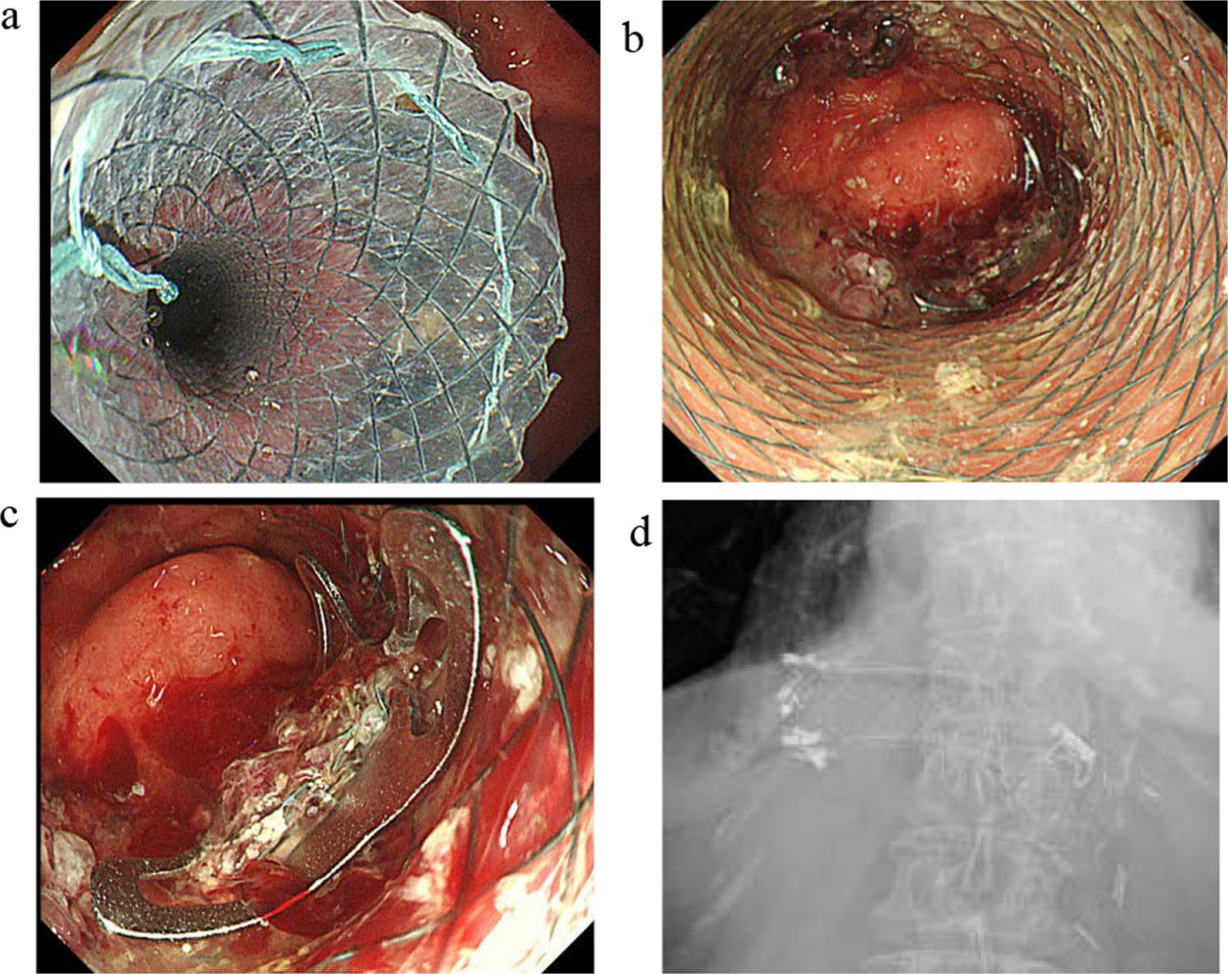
Endoscopic stent-in-stent placement. a A stent was placed inside the previous stent. b Patency of the new stent was confirmed. c The stent was fixed with the previous stent using OTSCs. d On X-ray, the new stent is patent. *OTSC* over-the-scope clip
